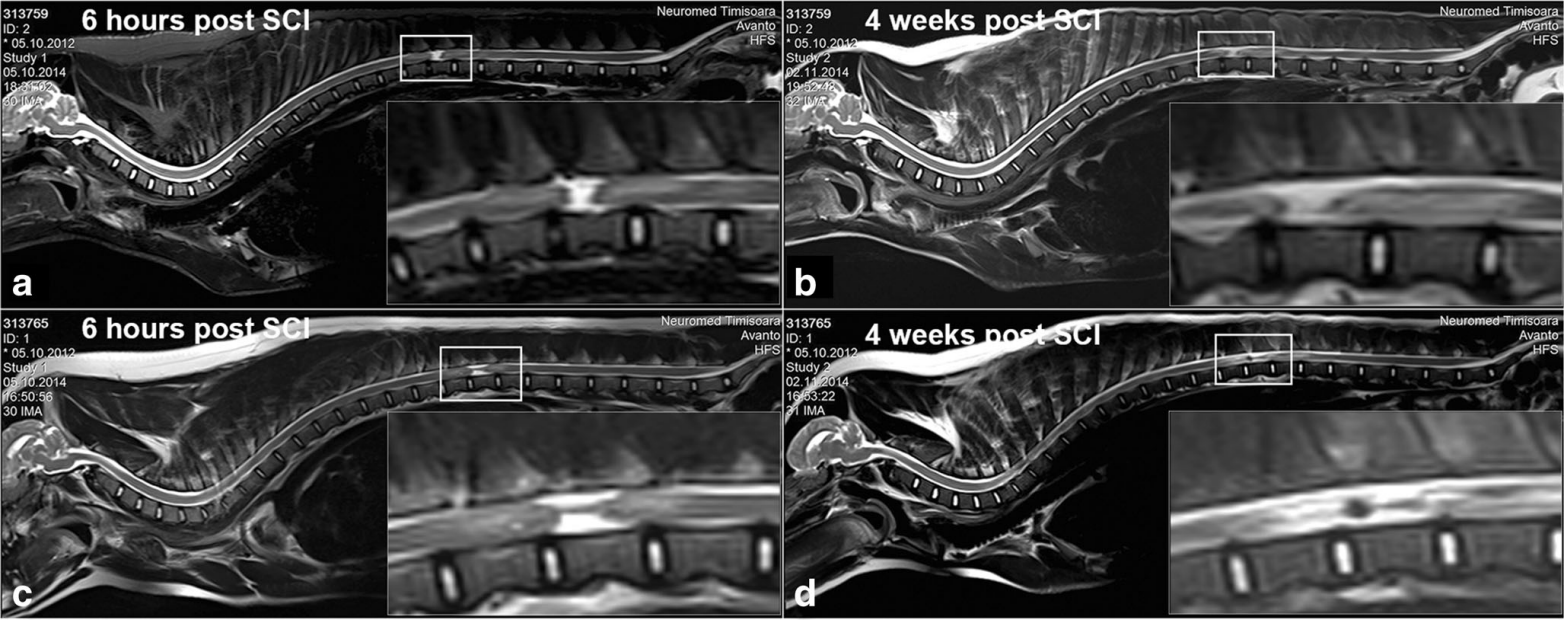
Magnetic resonance imaging (MRI) of the spinal cord of spinal cord injury (SCI) minipigs. a, c Complete spine MRI’s 6-h post SCI. The selected rectangle shows the lesioned region in higher magnification (lower right corner). b, d Complete spine MRI’s 4 weeks post SCI. The selected rectangle shows the lesioned region in higher magnification (lower right corner)
